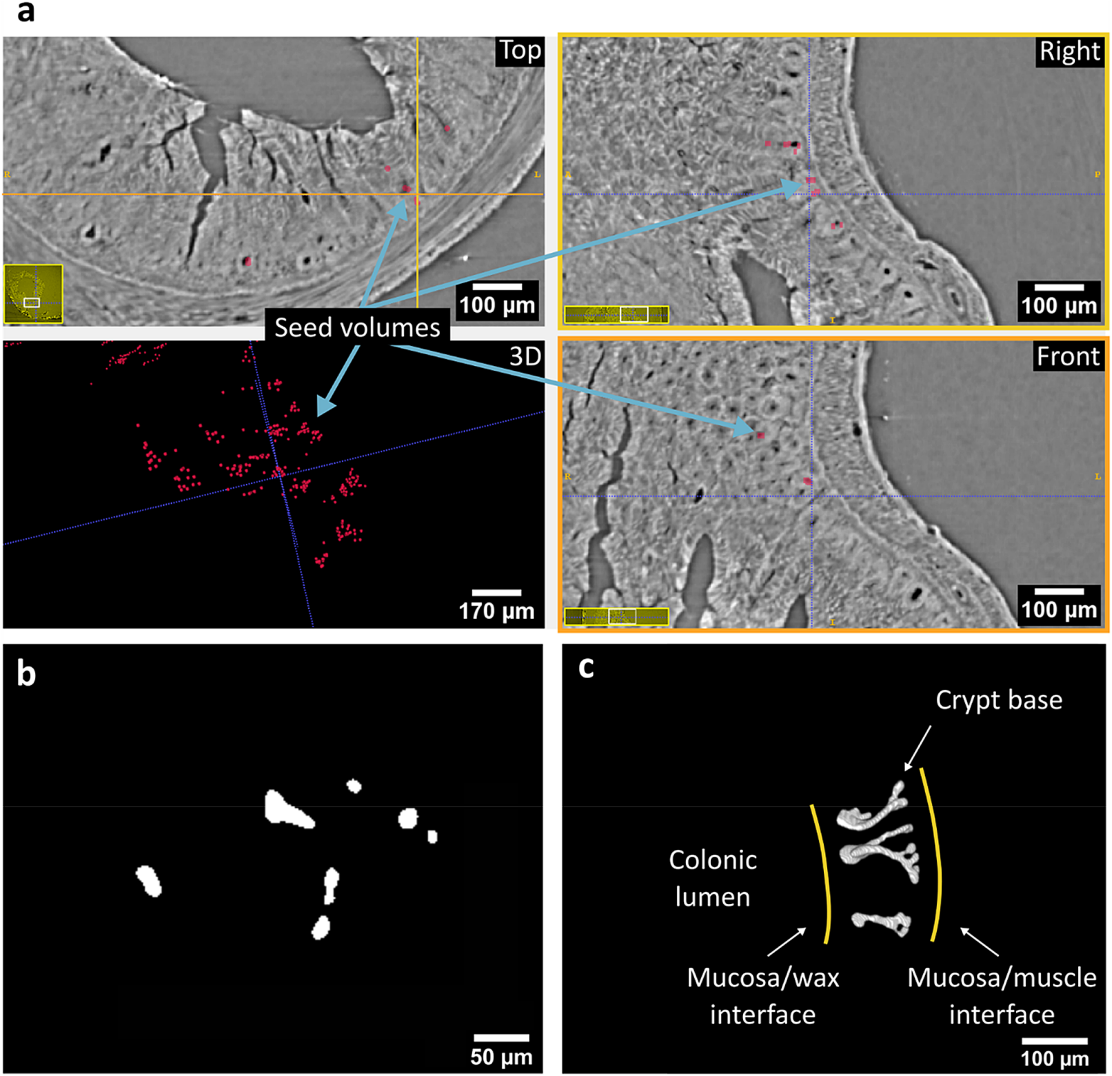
Budding crypt identification and 3D segmentation. (a) The viewport of ITK-SNAP is composed of four views (Top, Right, Front and 3D). The panels Top, Right and Front show orthogonal cross sections of the SR CT stack. The panels Right and Front show cross sections across the yellow and orange lines on the panel named Top, respectively. The panel named 3D shows the CT mask with the volumes of the back-mapped seed points (resulting from the inverse mapping between the 3D cyclorama and the CT data). The 3D seed volumes shown in the panel 3D, which are overlaid on the CT stack in the panels named Top, Right, and Front, are used to initialise the segmentation and expand it throughout the crypt lumens using region growing. The panels named Top, Right, and Front only show a zoomed-in view of the respective orthogonal cross sections. The white rectangles within the yellow panes on the bottom left corners of the Top, Right, and Front panels show which part of the cross sections is displayed in the panel. (b) Each slice of the image stack produced by the segmentation procedure is a binary image, with pixels corresponding to the crypt lumens having the value of one (white) and zero (black) elsewhere. (c) A 3D rendering of the binary image stack reveals the budding crypts and their position in space within the tissue.
